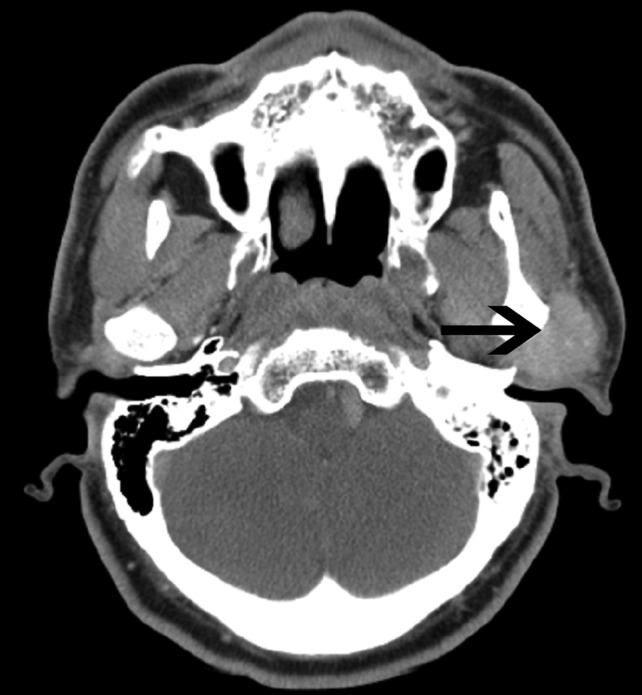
Computed tomography scan (axial view) of a mass, indicated by the arrow, identified in the parotid gland.
